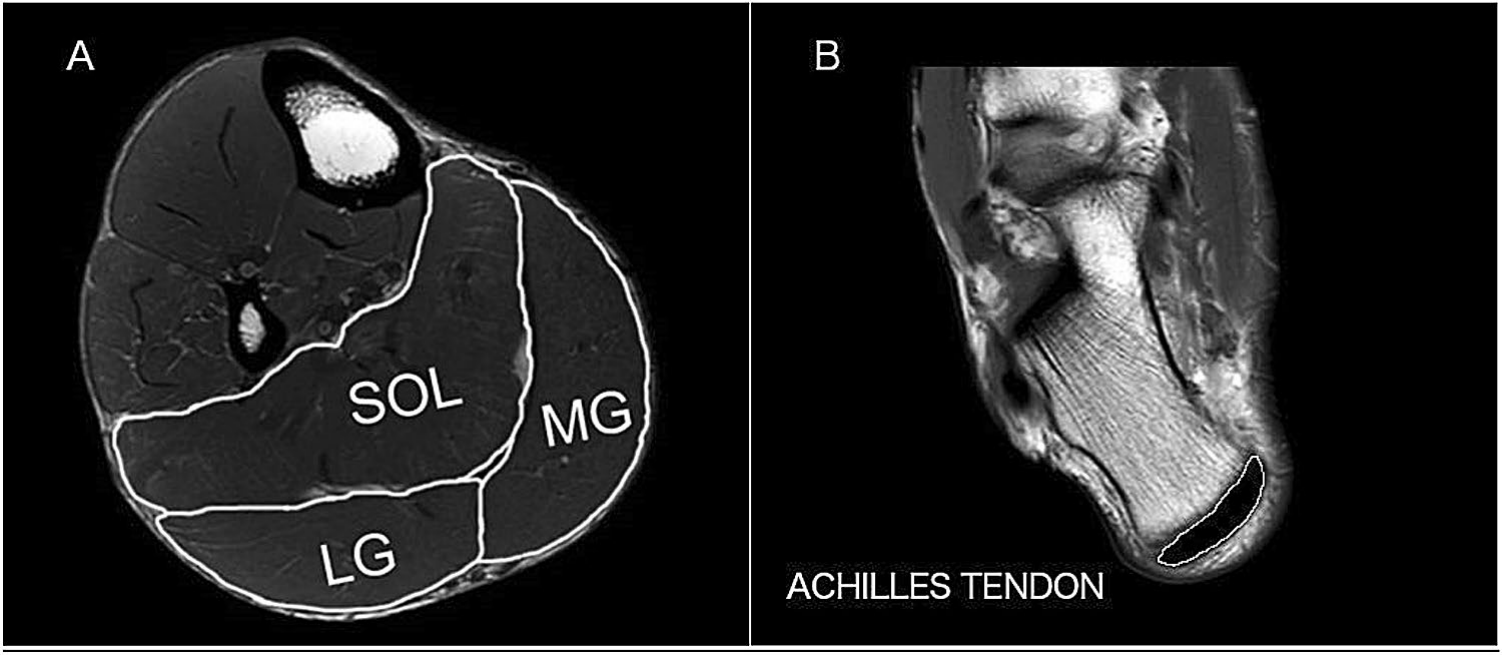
Representative magnetic resonance image from the middle of the lower leg for the calculation cross-sectional areas (A). The triceps surae compartments were separately outlined manually. (B) A sample image of the maximal distal Achilles tendon. Each segmented area (*SOL* soleus, *MG* medial gastrocnemius, *LG* lateral gastrocnemius) marked with white line.
